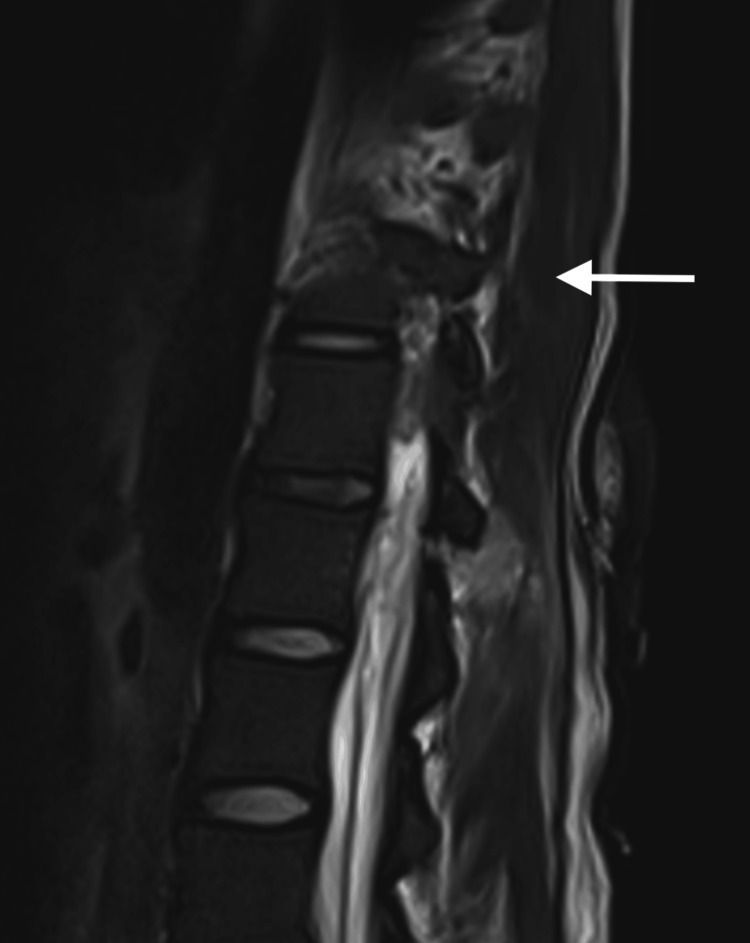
MRI T2 sagittal view of the stab at the L1 level
